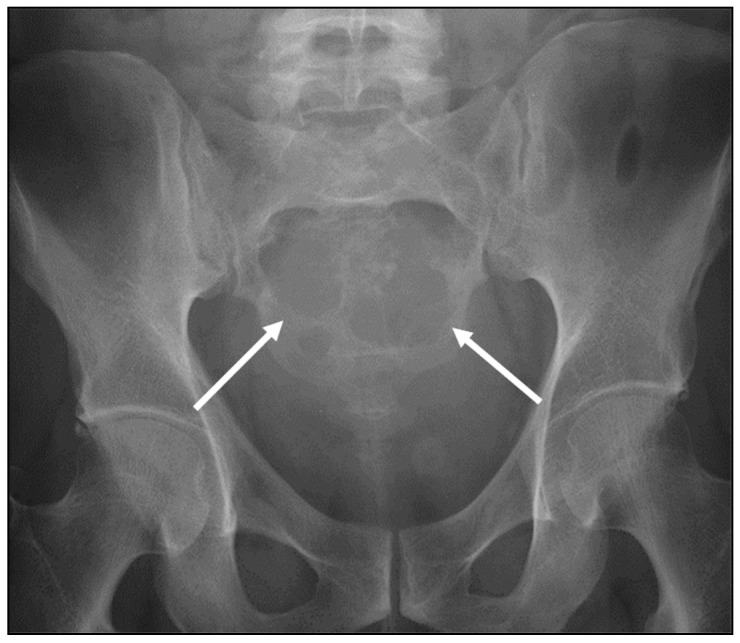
AP pelvic radiograph of a patient with sacral chordoma demonstrating the osseous destruction of the sacrum (white arrows).
